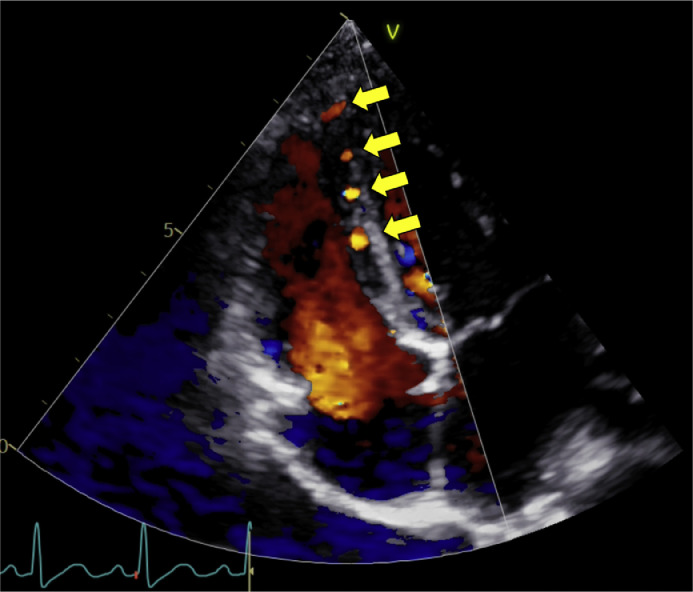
Echocardiography showed the characteristic Christmas tree pattern of anomalous left coronary artery from the pulmonary artery (yellow arrows).
